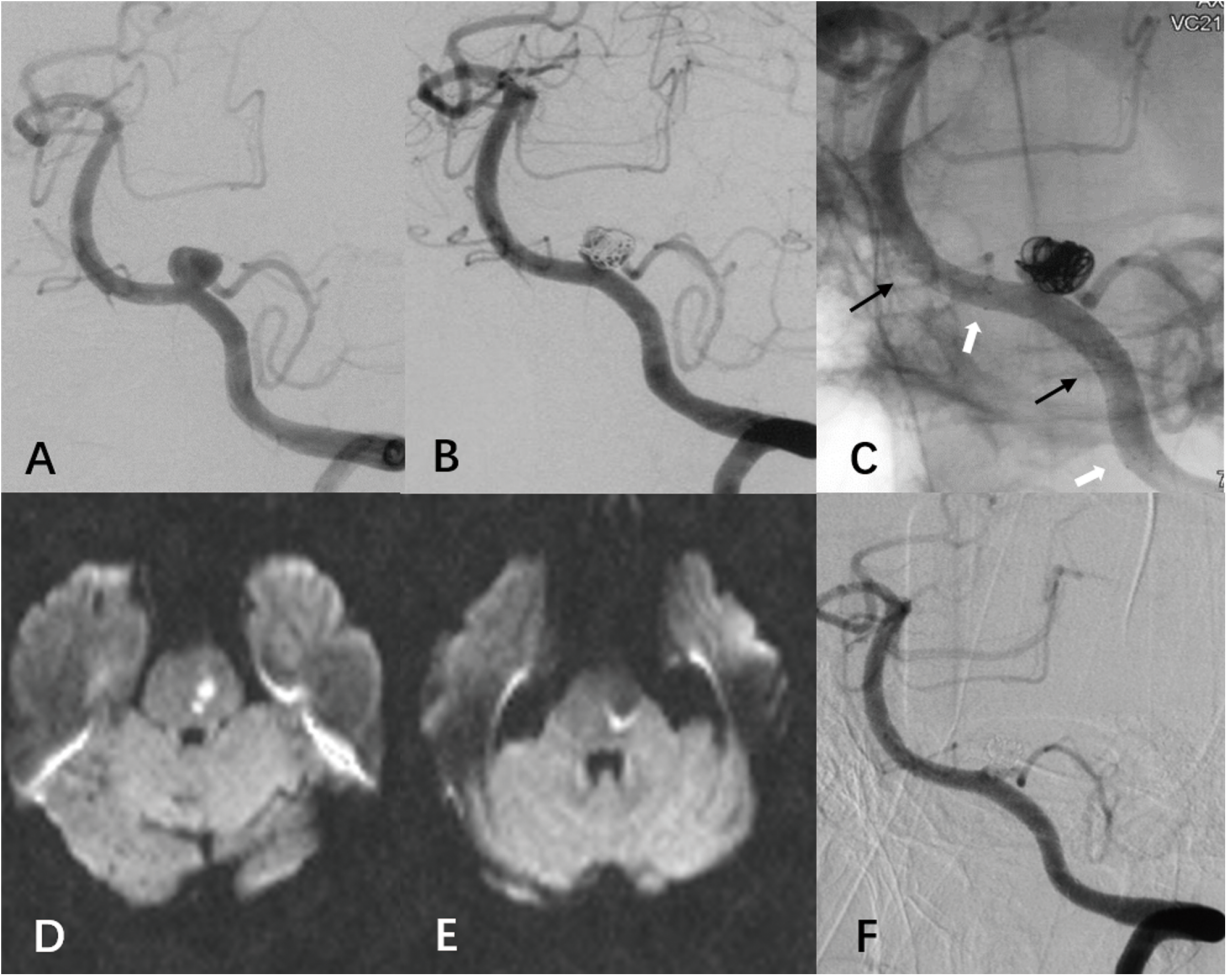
(case 22) The left vertebral angiography at admission showed a PICA-involved VADA, with the PICA arising from the lesion (A). We performed double overlapping LVIS stents (black arrow and white arrow) and loose coiling packing. The immediate angiographic result was partial occlusion of the VADA with the patency of involved PICA (B, C). This patient suffered from mild vertigo and hoarseness after treatment and MR imaging revealed two tiny punctate foci of diffusion restriction in the lower pon(D, E). The angiographic follow-up at 8 months showed progressive aneurysm occlusion without compromise of PICA (F).
